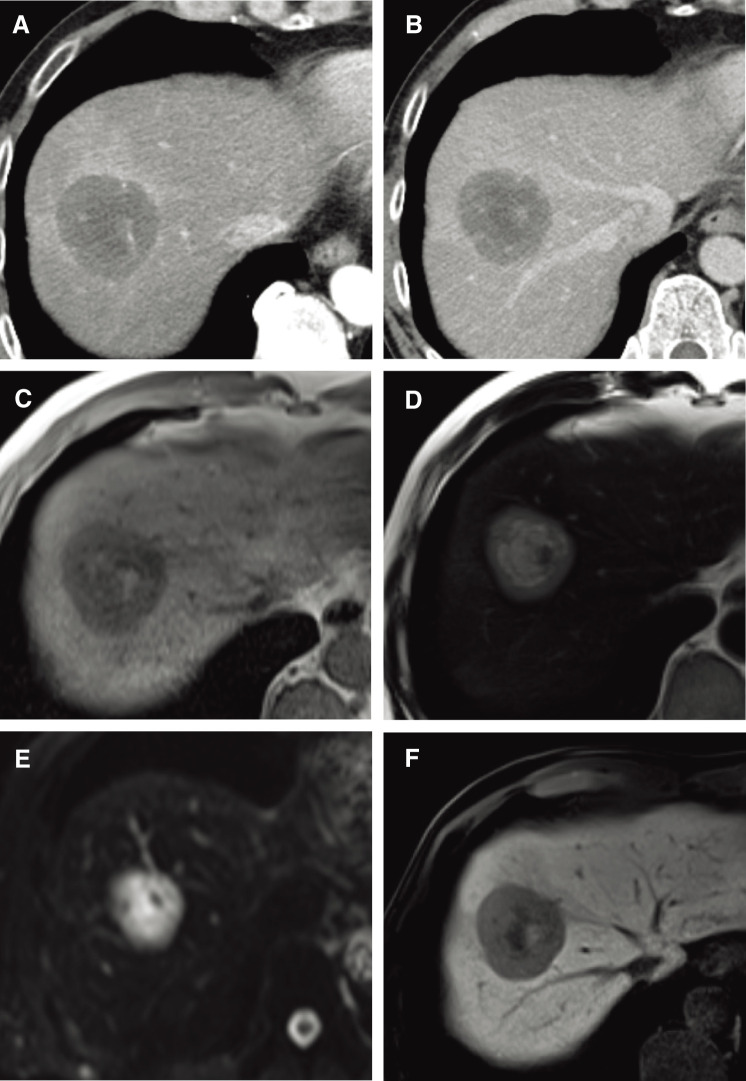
Imaging findings of primary HEHE. (A) Arterial phase of CT. (B) Portal phase of CT. (C) T1-weighted imaging of MRI. (D) T2-weighted imaging of MRI. (E) Diffusion weighted imaging of MRI. (F) Hepatobiliary phase of EOB-MRI. CT, computed tomography; EOB-MRI, ethoxybenzyl-diethylene-triaminepentaacetic acid-enhanced MRI; HEHE, hepatic epithelioid hemangioendothelioma; MRI, magnetic resonance imaging
